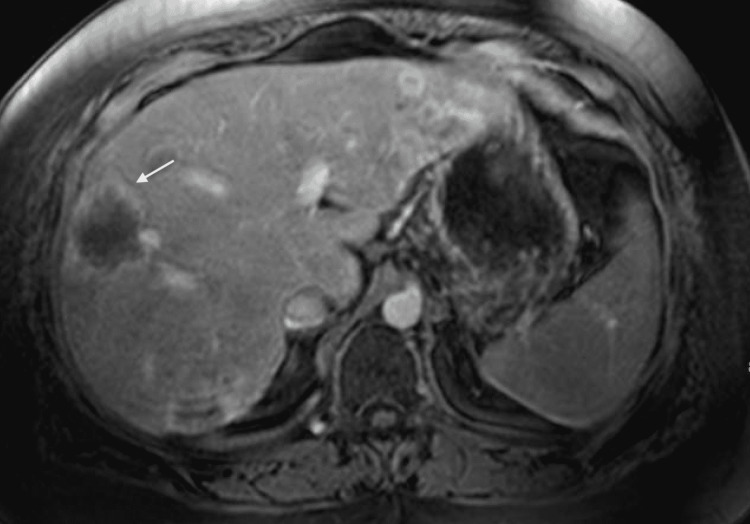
Axial view of an MRI abdomen T-1 weighted imaging taken one minute post contrast showing a hepatic lesion with peripheral enhancement (white arrow) MRI: Magnetic Resonance Imaging
